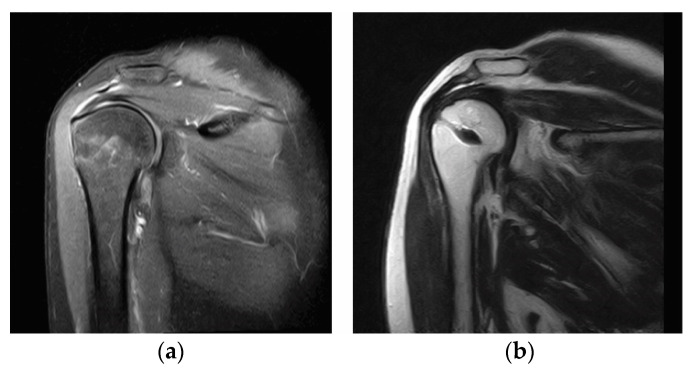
(a) Coronal MRI showing a partial tear involving the bursal side of the supraspinatus tendon. (b) Coronal MRI showing tendon healing after tear repair with the tear completion repair (TCR) technique. MRI, magnetic resonance imaging; TCR, tear completion repair.
